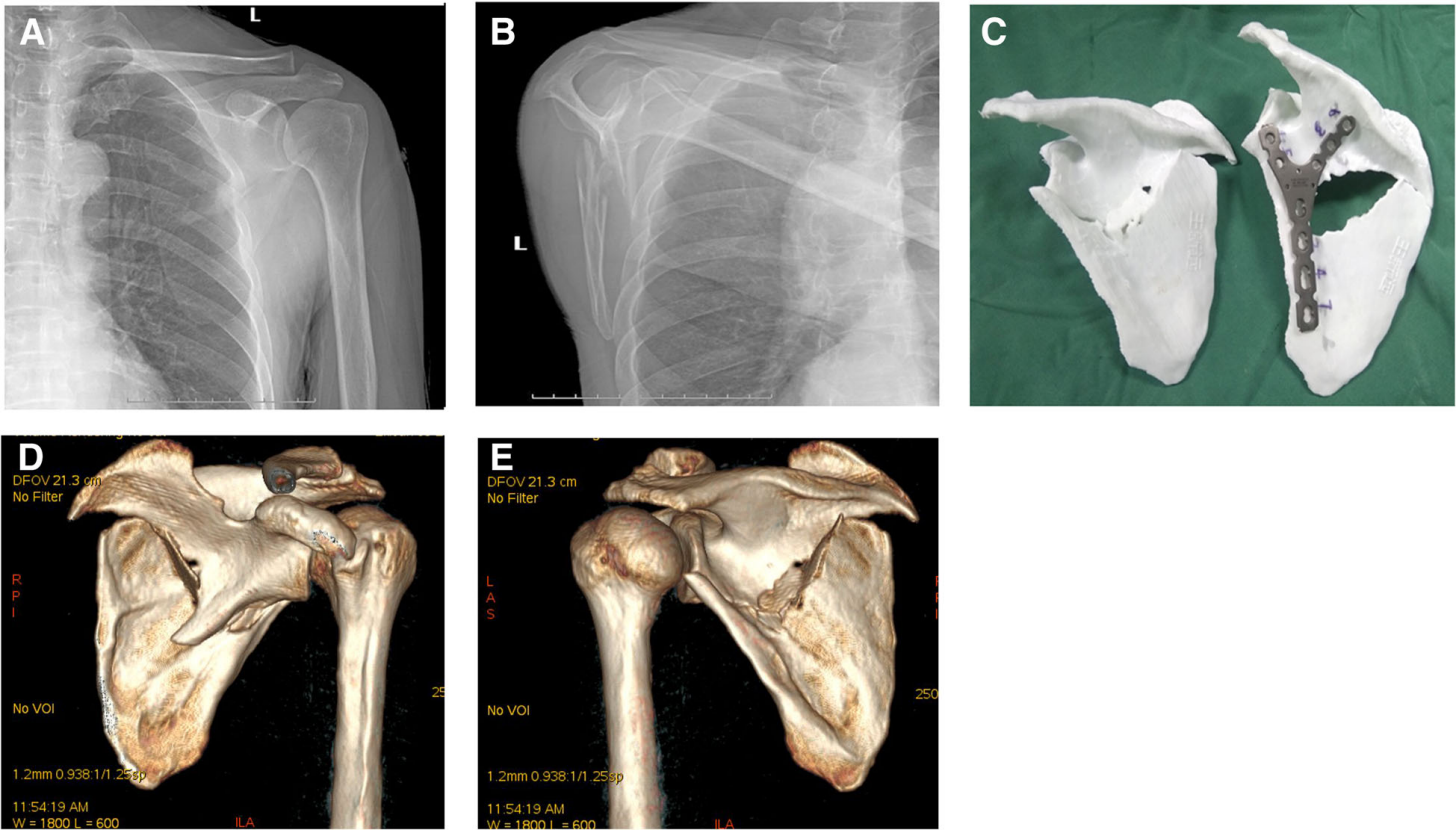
A 61-year-old man sustained a left scapular body and glenoid neck fracture (AO 14-A3.1) associated with multiple left rib fractures. a GPA was 26.4° on the anteroposterior X-ray film of the scapula fracture. b The lateral displacement was 33.2 mm (defined as displacement between the proximal and distal fragments at the lateral border) and angular deformity was 34.3° on the lateral X-ray film. c 3D model, fracture reduction, and preoperative repair planning. d–e Three-dimensional computed tomography reconstruction of the scapula fracture
